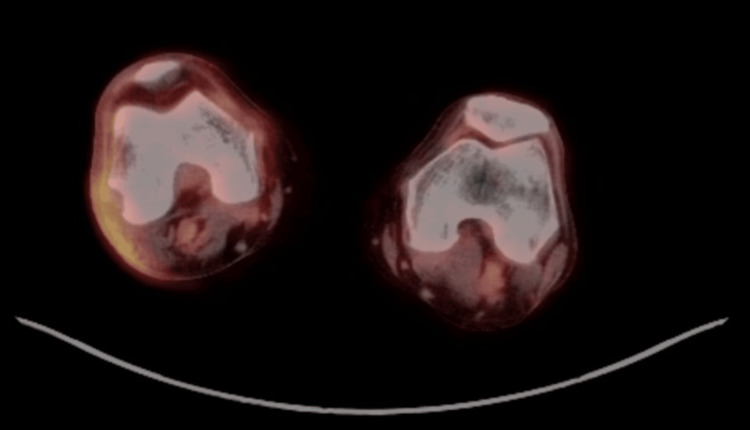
PET-CT findings 18F-FDG PET-CT demonstrating hypermetabolic uptake corresponding to the ulcerated cutaneous lesion of the right distal thigh, associated with ipsilateral popliteal and inguinal lymph nodes showing reactive metabolic activity without evidence of distant systemic disease. 18F-FDG PET-CT: 18-fluorodeoxyglucose positron emission tomography-computed tomography
